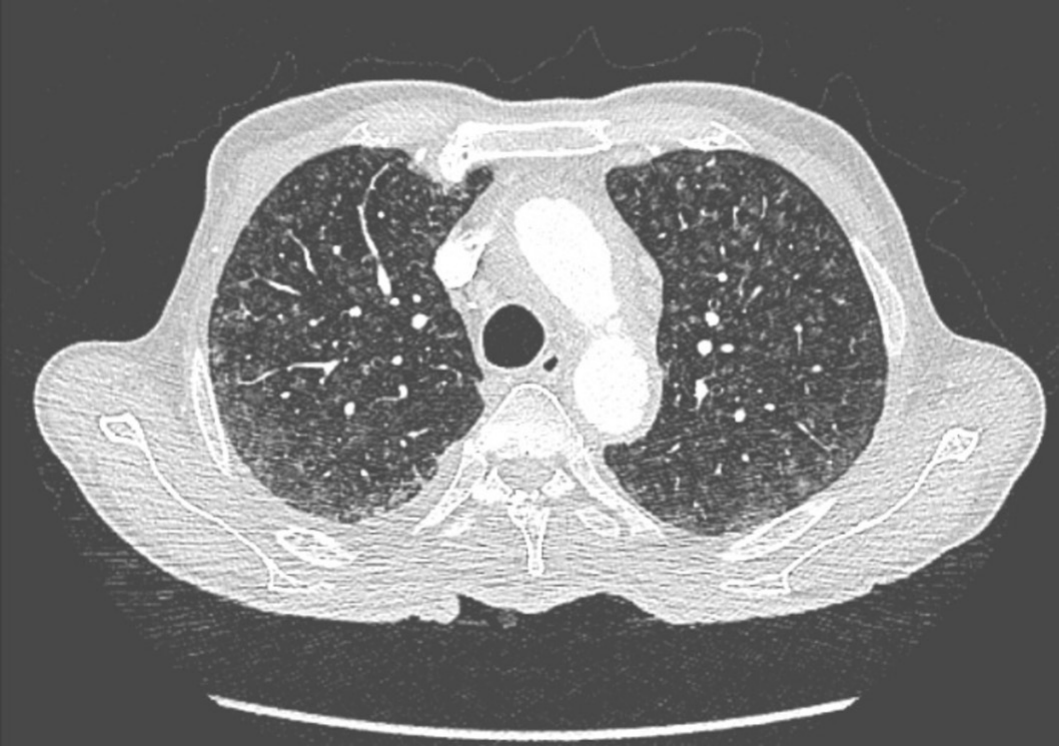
High‐resolution computed tomography (HRCT) of the chest showing bilateral milliary pattern with centrilobular nodules.
